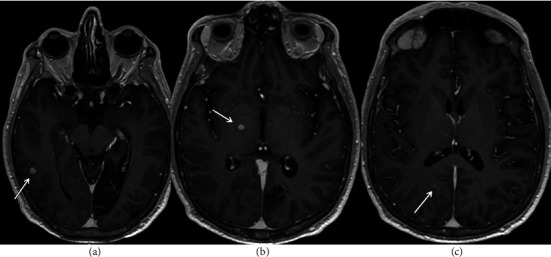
MRI brain with and without contrast showing numerous enhancing lesions.
